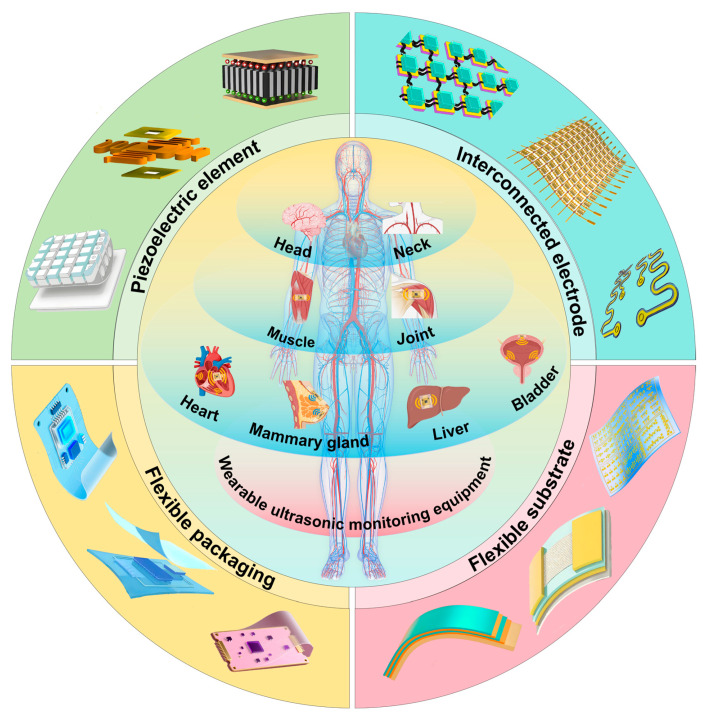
The structural design of wearable ultrasonic transducers and the application of wearable ultrasonic monitoring devices on the human body.
